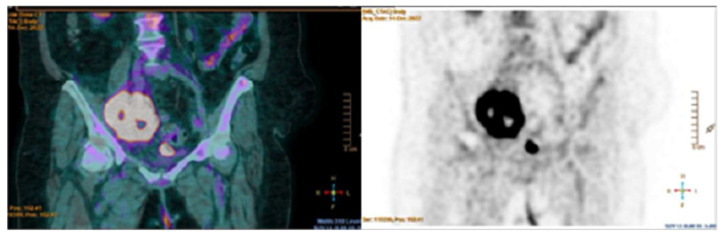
A large mass in the right pelvic region exhibiting widespread and non-uniform ^18^F-FDG on the PET/CT scan.
